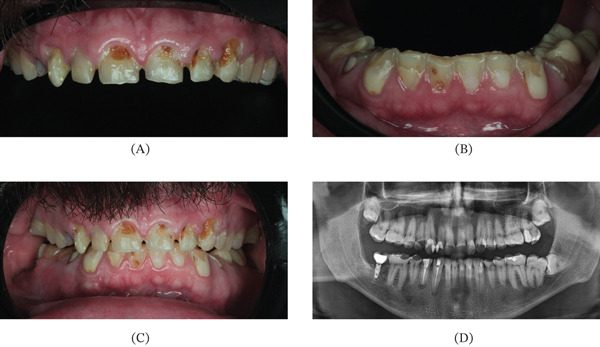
Initial clinical status. (A) Maxillary frontal view. (B) Mandibular frontal view. (C) Intraoral frontal view. (D) Initial panoramic radiograph.
